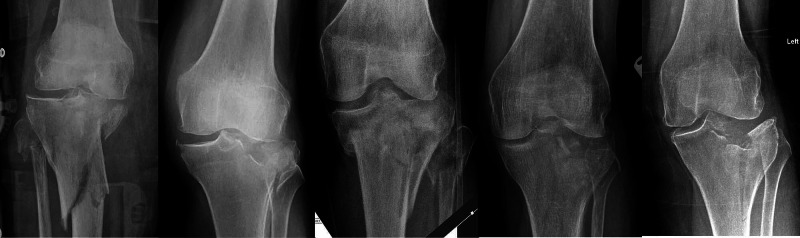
Anterior-posterior radiographs on the day of the injury (Case 1 to 5, left to right)
